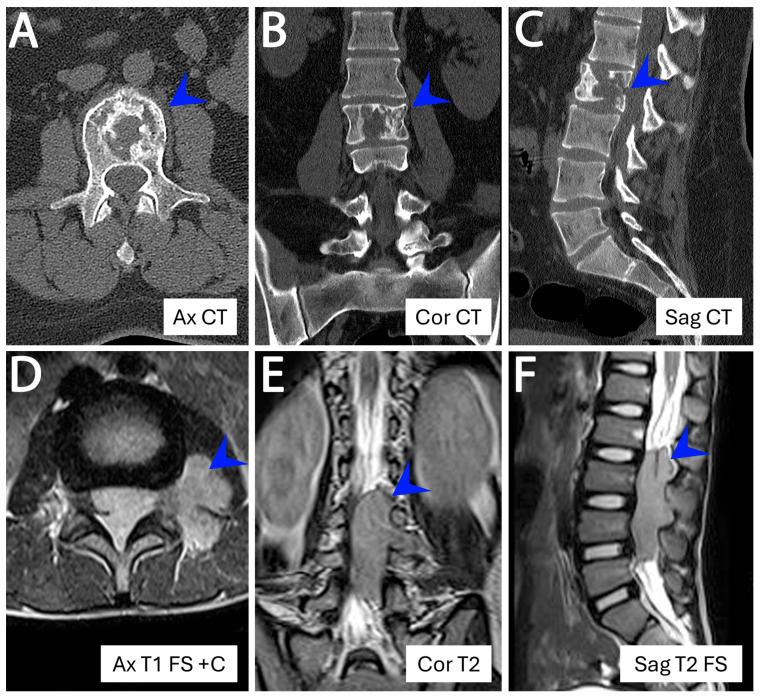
Contrasting two *EWSR1::FLI1*–positive Ewing sarcomas of the spinal region. (A–C) Skeletal tumor arising from the L2 vertebral body in a 24-year-old man. (D–F) Extraskeletal extradural tumor in the paraspinal soft tissue with foraminal extension and infiltration of surrounding muscles, in an 8-year-old boy. Ax = axial; Cor = coronal; Sag = sagittal; CT = computed tomography; T1 = T1-weighted MRI; T2 = T2-weighted MRI; FS = fat saturation; C = contrast.
